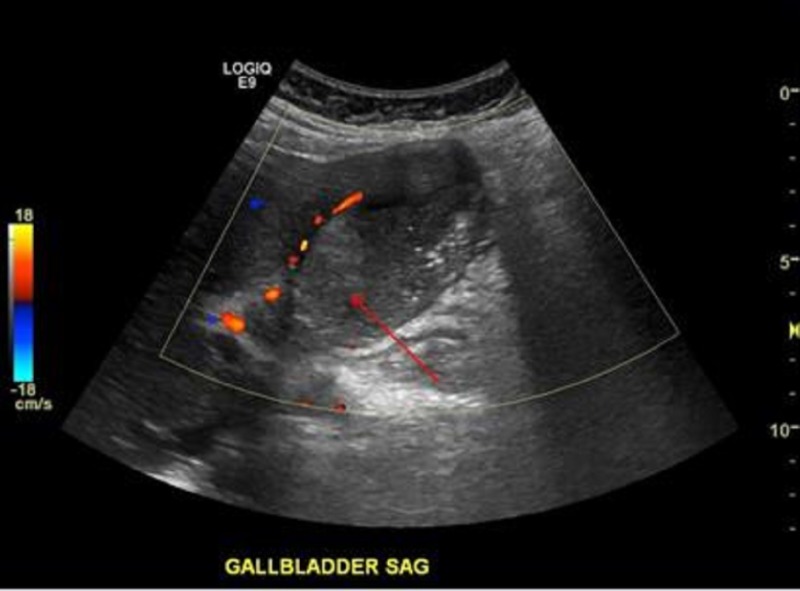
Right upper quadrant ultrasound. The image demonstrates sludge/stone like material within the lumen of the gallbladder (red arrow) with a rim of hyperemia initially interpreted as outside of the gallbladder given the clinical appearance of acute cholecystitis.
